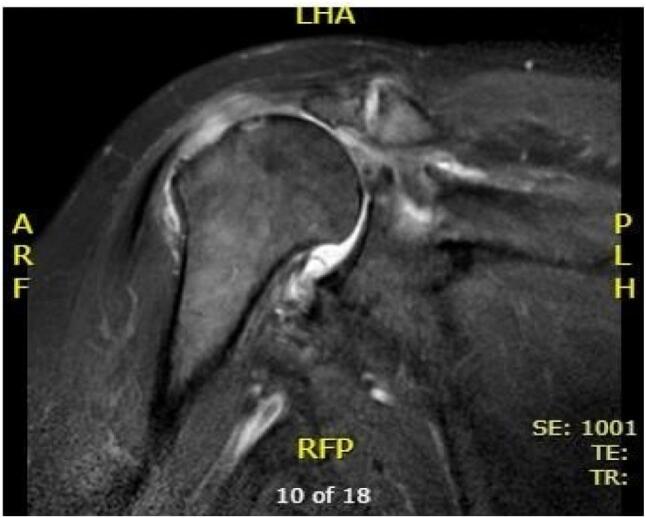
Magnetic resonant image (MRI) showing rotator cuff tear with medial retraction.
